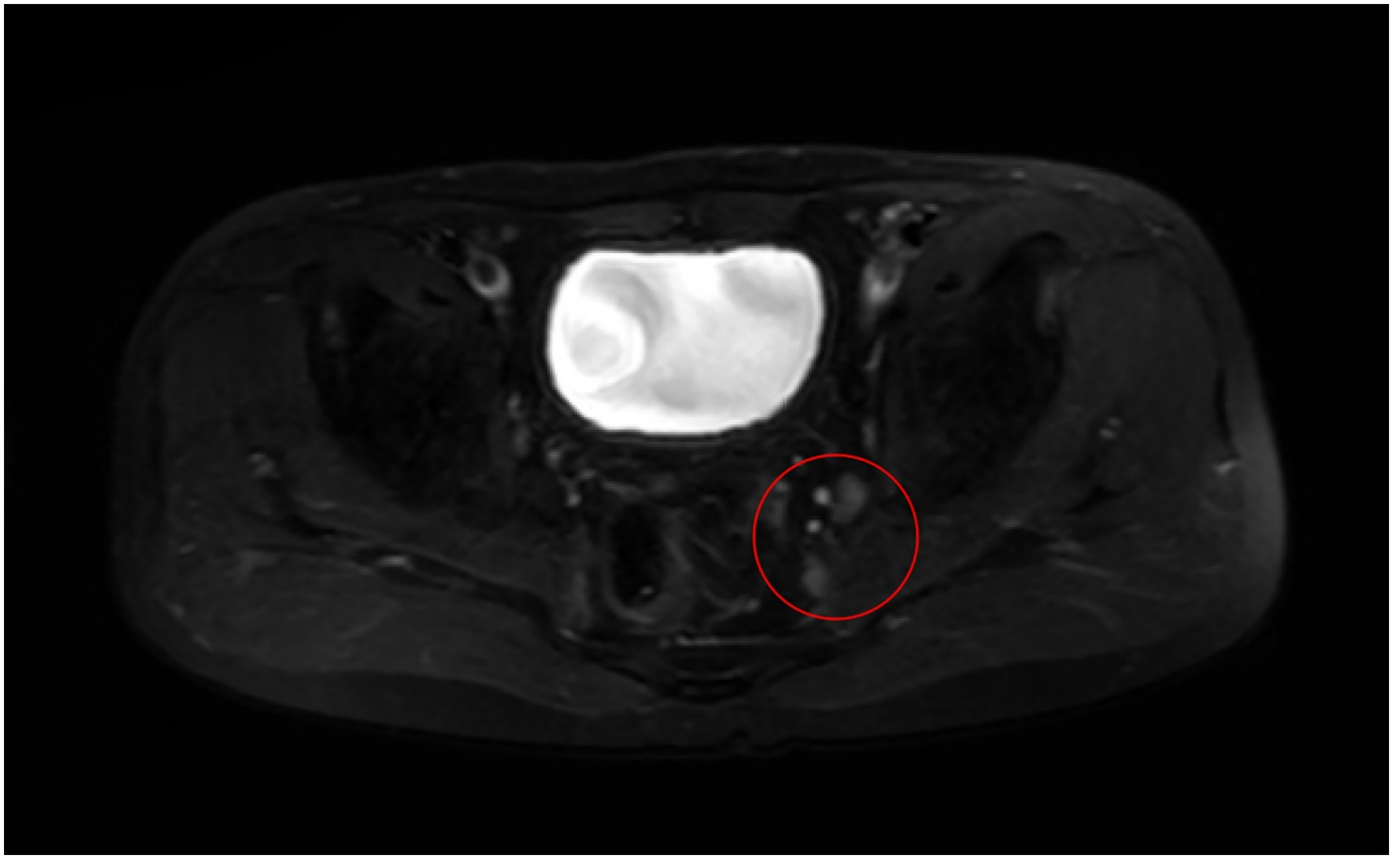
Preoperative MRI showed a short diameter of the lateral lymph node of the rectum of ≥10 mm.
